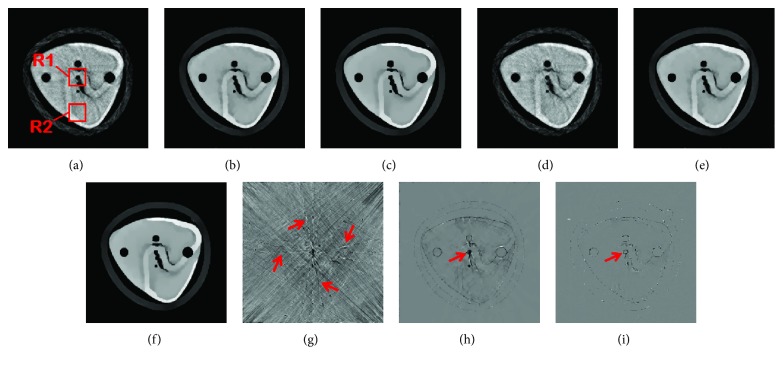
CT images from SART (a, d, g), TV (b, e, h), and TF-L0 (c, f, i). Images (a), (b), and (c) are reconstructed from the ideal projections. Images (d), (e), and (f) are reconstructed from the interpolated projections. The third row images are the images showing the differences between images in the first and the second rows.
